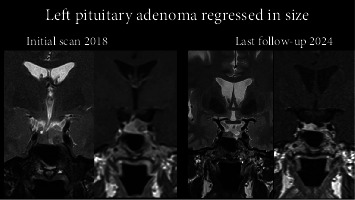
MRI before (left), and after (right) pasireotide therapy showing reduction in size of the pituitary adenoma.
